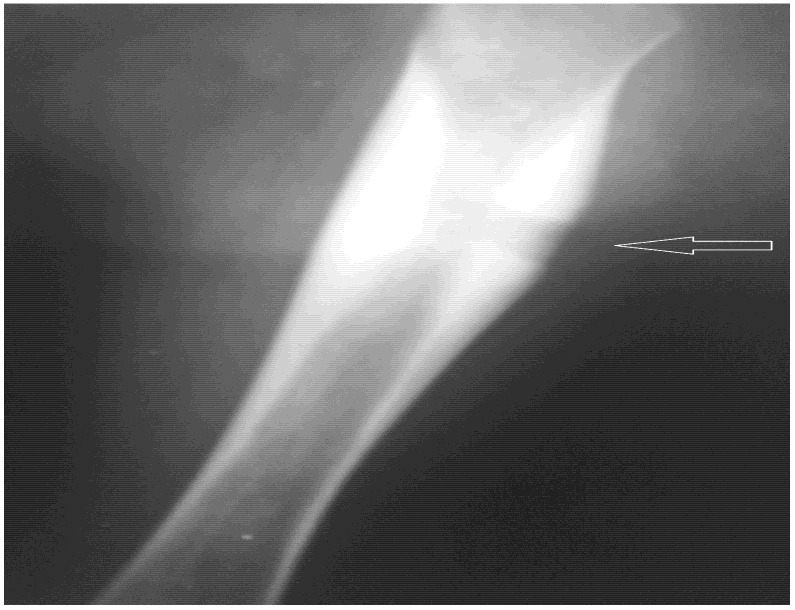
Postoperative radiograph demonstrating the cortical window and open medullary canal.
